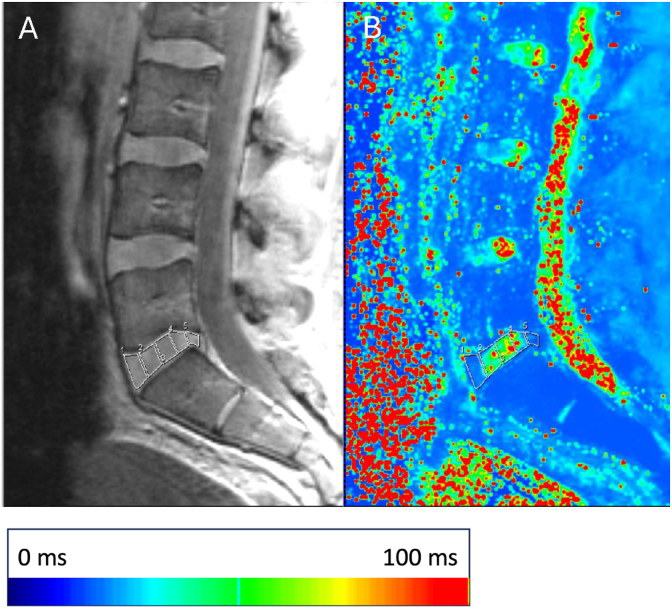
(A) Midsagittal reformat of T2∗-weighted morphological magnetic resonance imaging and (B) the corresponding midsagittal T2∗ map. Five regions of interest were identified in the sagittal direction: anterior annulus fibrosus (AF), anterior nucleus pulposus (NP), central NP, posterior NP and posterior AF. Note: The observer tried to create 5 zones manually, that reflected the anatomy of the IVD and were roughly proportioned in the sagittal plane. However due to the manual creation, the ROIs were not equal in size, neither between nor within a single IVD level. In addition, the disk shape is not rectangular and exhibits variations in height in the sagittal course. To ensure a reliable measurement of tissue within the IVD, the ROIs did not strictly adhere to the exact contours of the IVD. As a result, a few pixels of potential IVD tissue may have been excluded from the ROIs.
